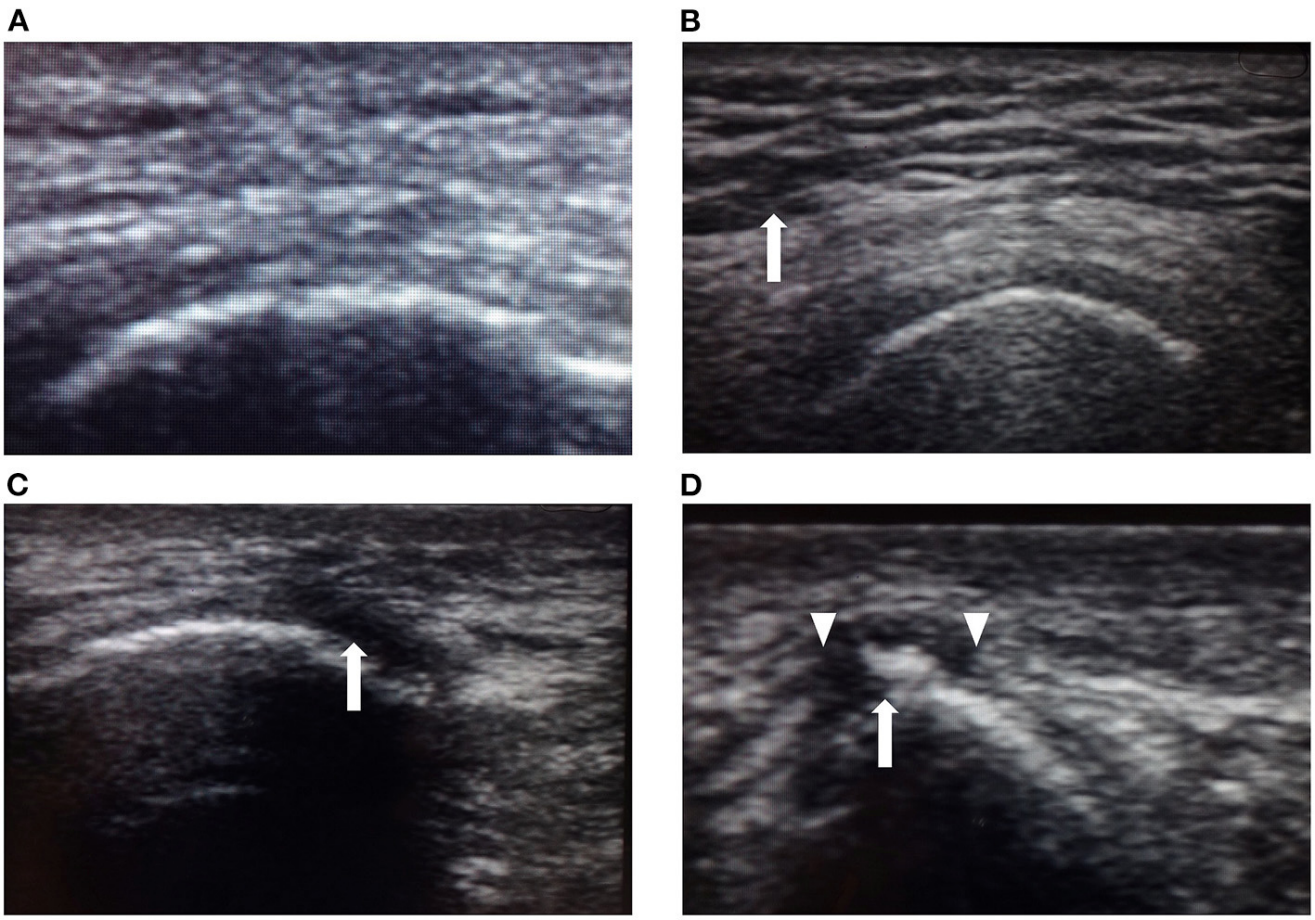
Typical ultrasound images. (A) Ultrasound image of normal skin showing a homogeneous pattern of ultrasonic reflections from the epidermis to the right ischial bone (arrow), with a clear layer of muscle. (B) Unclear layered structure with a coarse echo texture (arrow) is most frequently seen even in areas where hypoechoic lesions are not present and judged separately from low-echoic lesions. (C) Low-echoic lesion near the right ischial region in Case 2. The image shows a hypoechoic area near the bone (arrow). This hypoechoic area did not disappear when compressed. (D) Low-echoic lesion near the right ischial region in Case 7. Heterogeneous hypoechoic areas contained high-echoic areas (arrow). The image shows an unclear layered structure and discontinuous fascia. Discontinuous fascia shows a broken or discontinuous line with heterogeneous echo signals (triangle).
